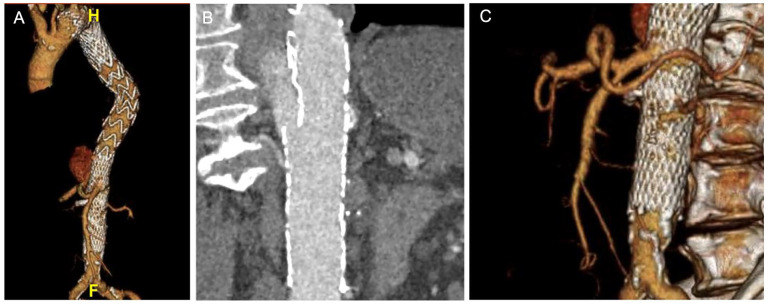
The preoperative computed tomography angiography confirmed the presence of a 68 mm type V thoracoabdominal aneurysm in a patient previously treated with thoracic endovascular repair and distal PETTICOAT for an acute complicated type B aortic dissection (Panel A). Potentially cause–effect relationship between aneurysm formation and bare metal stent fracture cannot be excluded (Panel B). The preoperative CTA also confirmed the presence of an anatomic variation with a common trunk for the celiac artery and superior mesenteric artery (Panel C). CTA, computed tomography angiography; PETTICOAT, Provisional Extension to Induce Complete Attachment Technique
